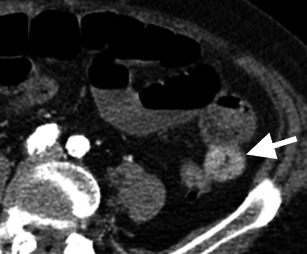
Bowel wall thickening and haemorrhage. Axial CT image in a patient with an occluded superior mesenteric artery. A short segment of jejunum shows circumferential thickening and hyperattenuation, favoured to represent bowel wall heamatoma
